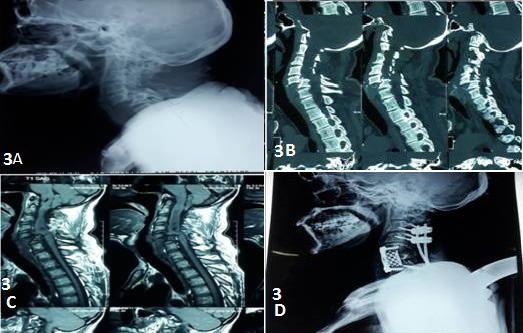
(A) Preoperative lateral X-ray of the cervical spine showing complete dislocation at the C5-6 level. (B) Preoperative CT scan of the cervical spine with complete bilateral facet dislocation C5-6 level. (C) Preoperative MRI showing significant cord compression at the C5-6 level. (D) Postoperative lateral x-ray showing anterior cervical plate and mesh cage with lateral mass screws and rod in situ.w
